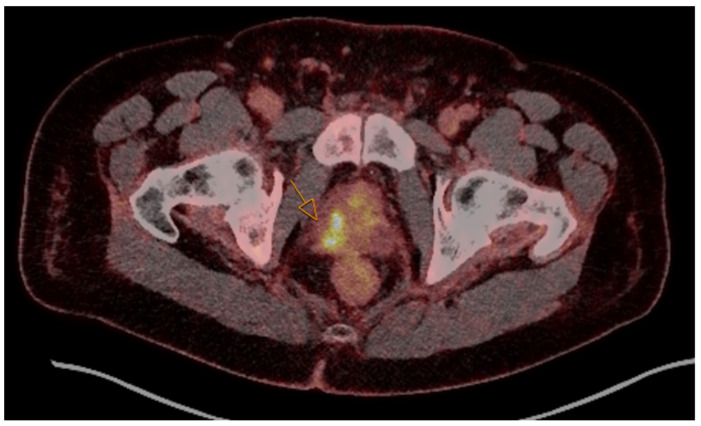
In this axial-fused PSMA PET/CT, the arrow shows a scapular focus of increased radiotracer uptake involving the right posterior peripheral zone of the base and mid gland with extension of malignancy in the base of the right seminal vesicle in a 74-year-old prostate cancer patient with hypertension and diabetes.
